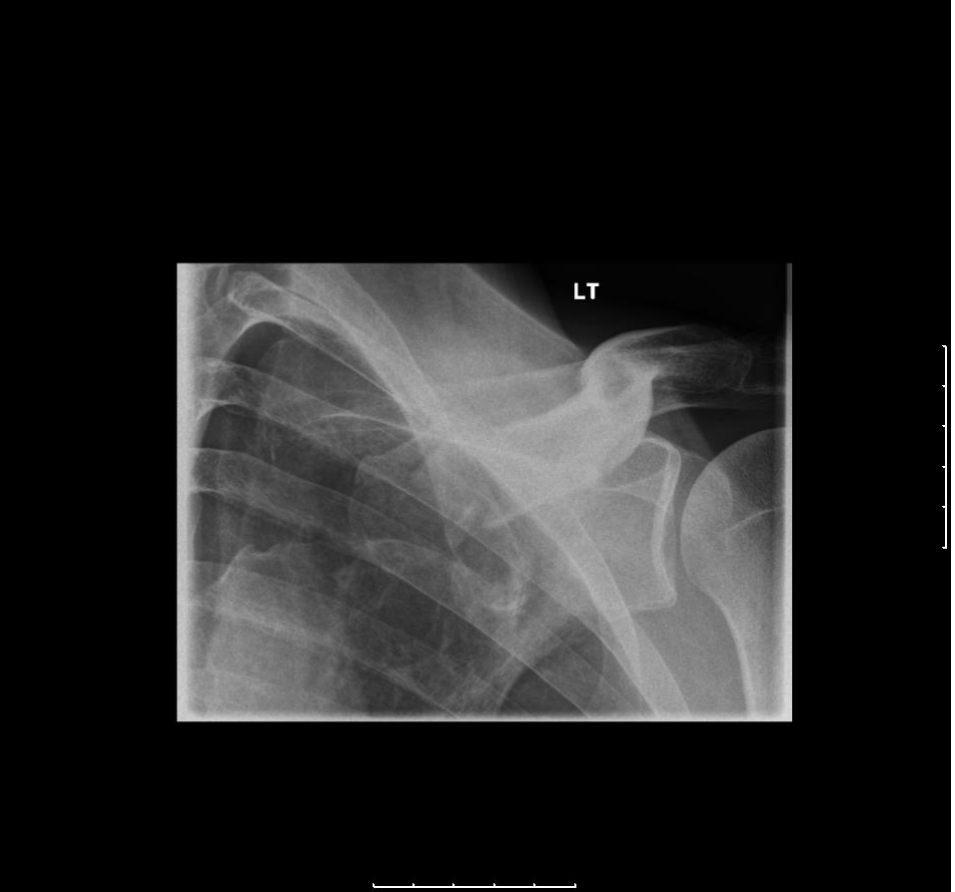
Oblique radiograph of the left clavicle demonstrating a fracture of the medial third (day 0).
